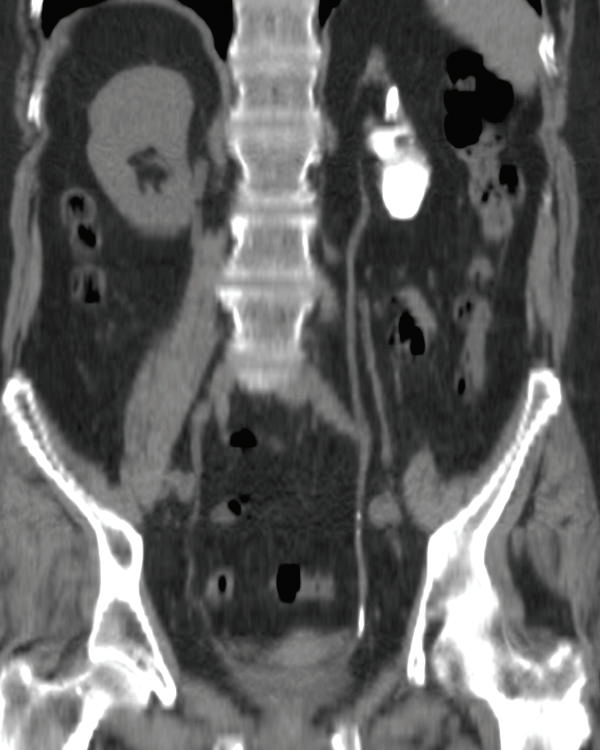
Non-contrast CT, coronal curves reformat.
